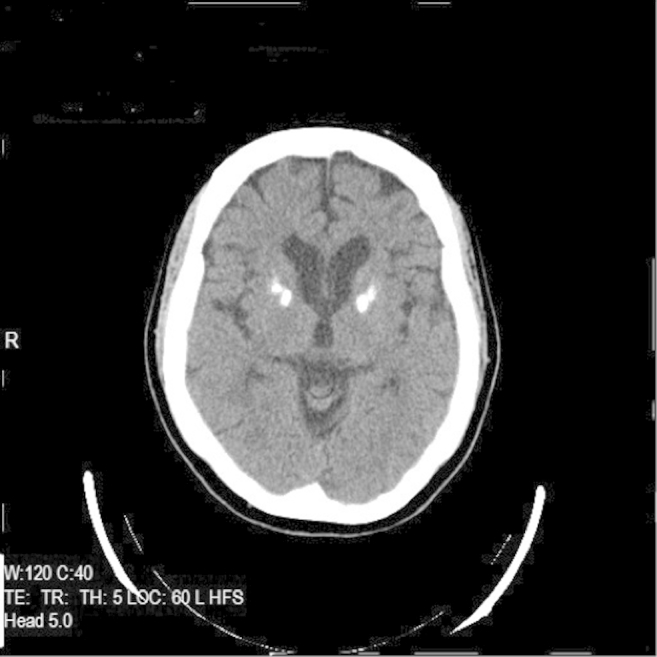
Computed tomography of the proband reveals symmetrical hyperdense lesions resembling calcifications in the globus pallidus.
